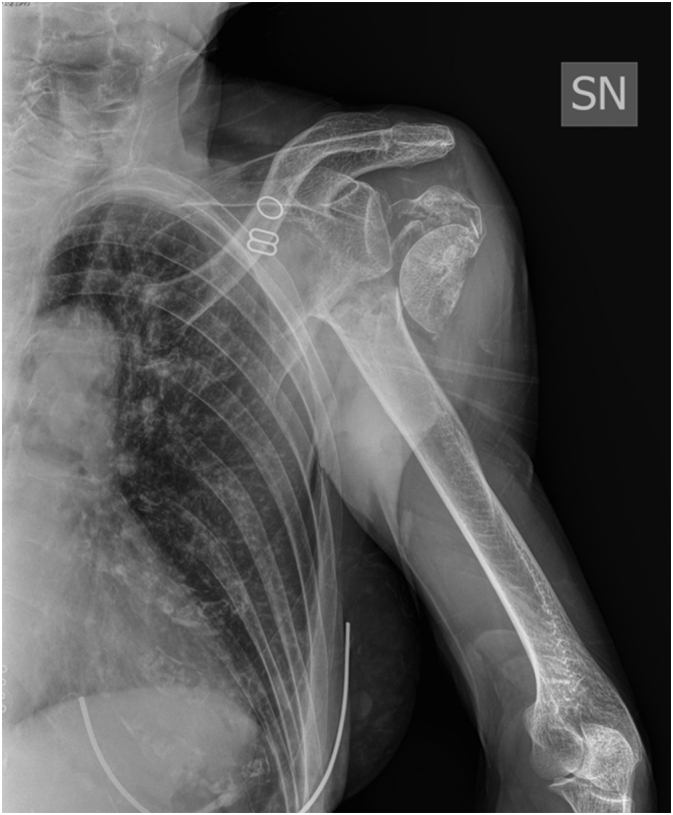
Plain X-ray at the admission.
